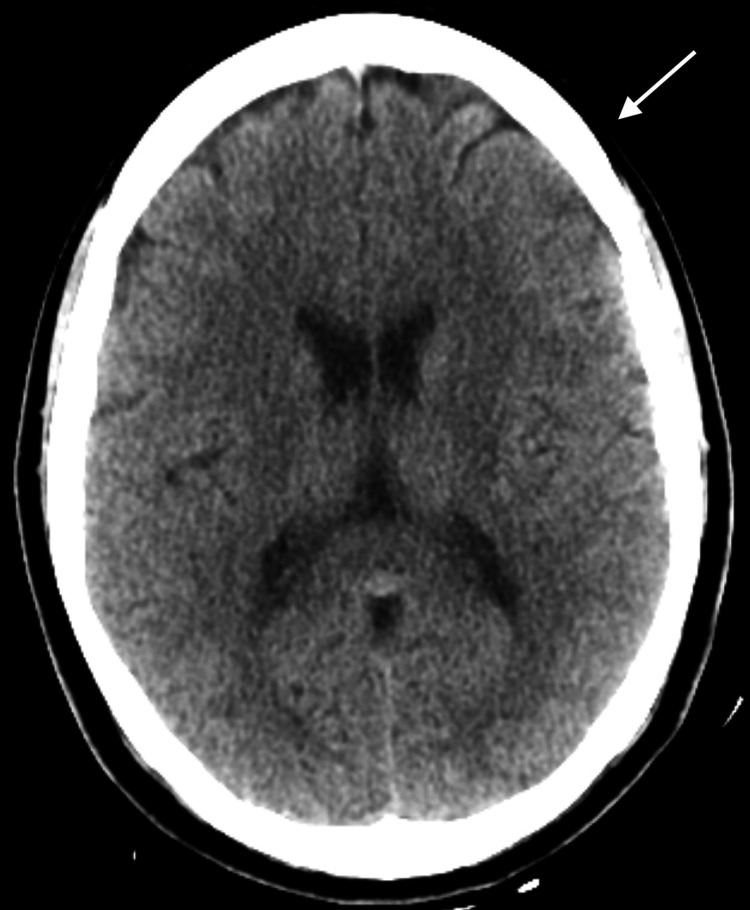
CT scan of the head (axial view) with normal ventricular size and configuration and no evidence of acute hemorrhage, ischemia, mass, mass effect, or midline displacement of structures CT: computed tomography
